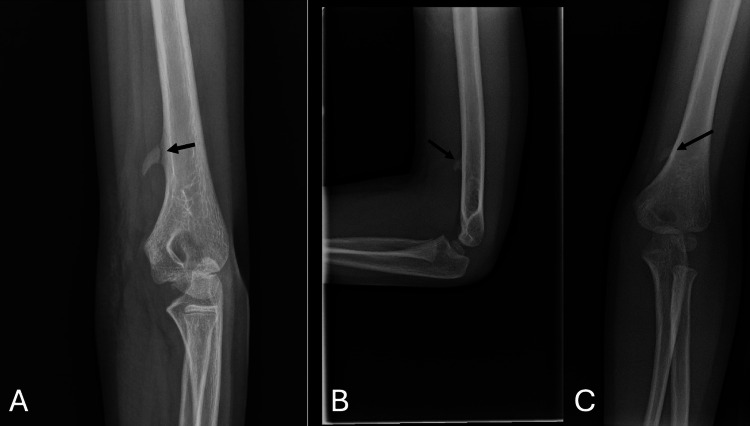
(A) Internal rotation oblique radiograph of left distal humerus in an 11-year-old male shows a supracondylar process with a fracture at its base. Note that the medial humeral cortex is continuous (arrow) so that the medullary canal of the supracondylar process does not continue into the medullary canal of the humerus. (B & C) Anteroposterior and lateral radiographs of the same patient at five years of age (arrows).
